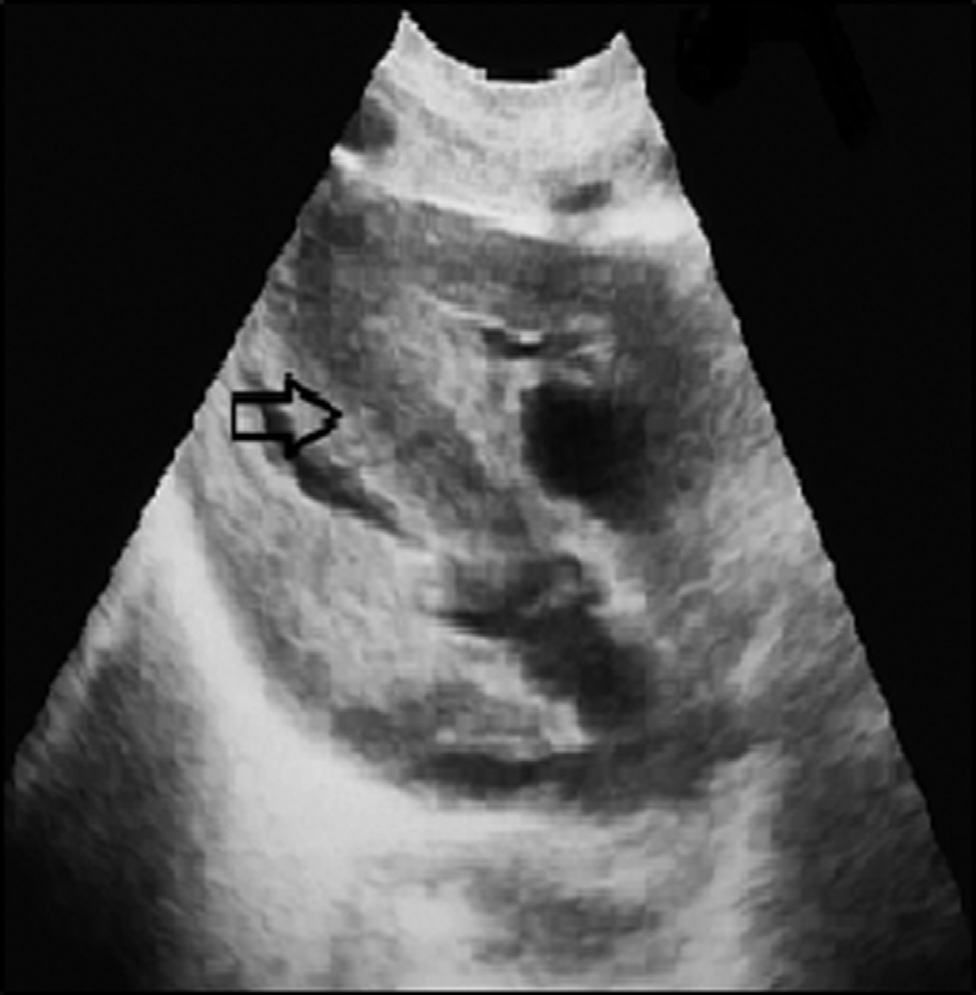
Echocardiography demonstrating asymmetrical hypertrophy of the interventricular septum. The arrow is an indication of the echocardiography of the patient showing asymmetric septal hypertrophy.
